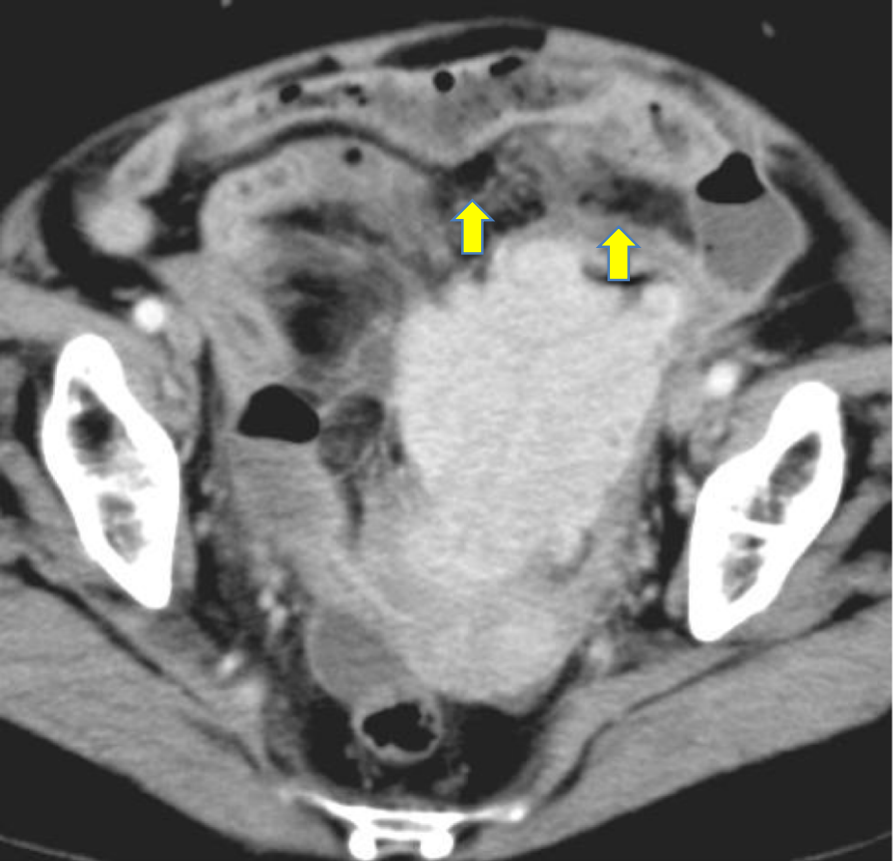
Plain abdominal computed tomography. Huge tumor is present at the sigmoid colon, and free air (arrows) is seen around the tumor
